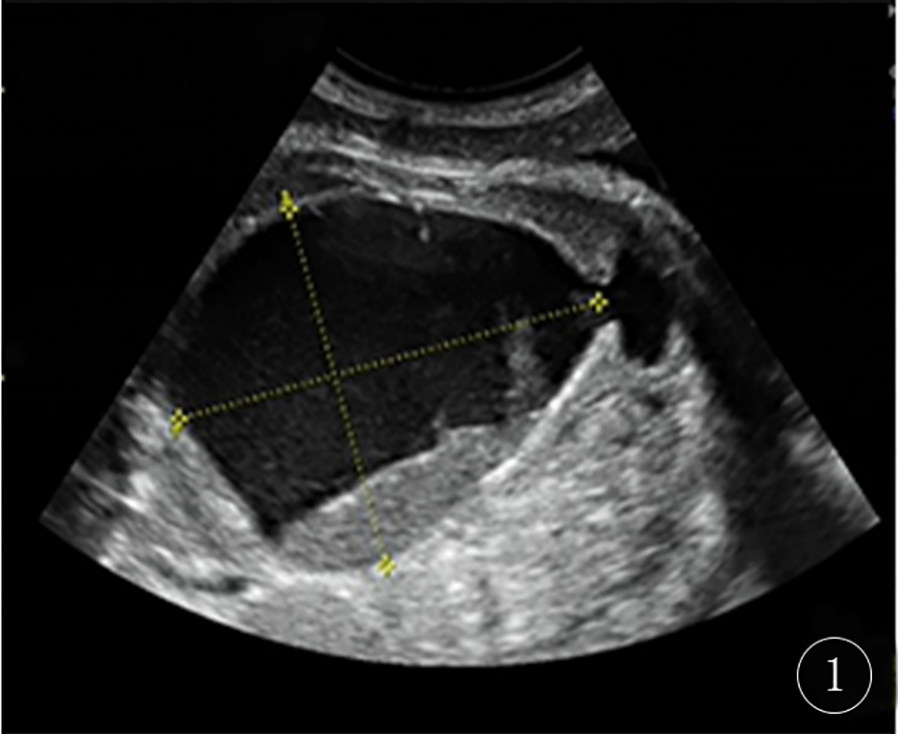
Fetal ultrasound at 37 weeks + 3 days the image shows a large cystic mass, approximately 64 mm × 83 mm in size, within the fetal pelvis. The cyst contents exhibit heterogeneous echogenicity.
